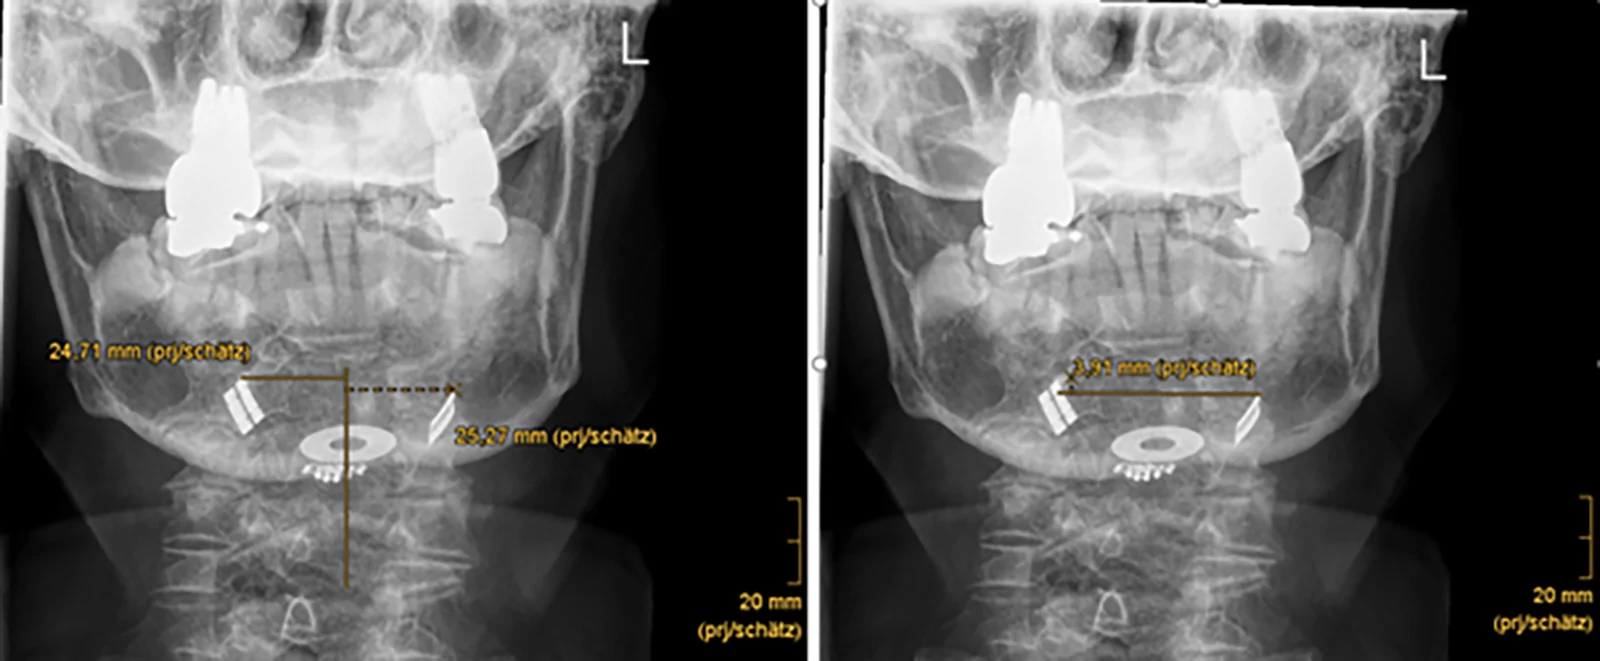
x-ray (a.p.). Quantitative analysis of implant position. Patient with symmetrical horizontal electrode position and vertical symmetrical electrode position.
